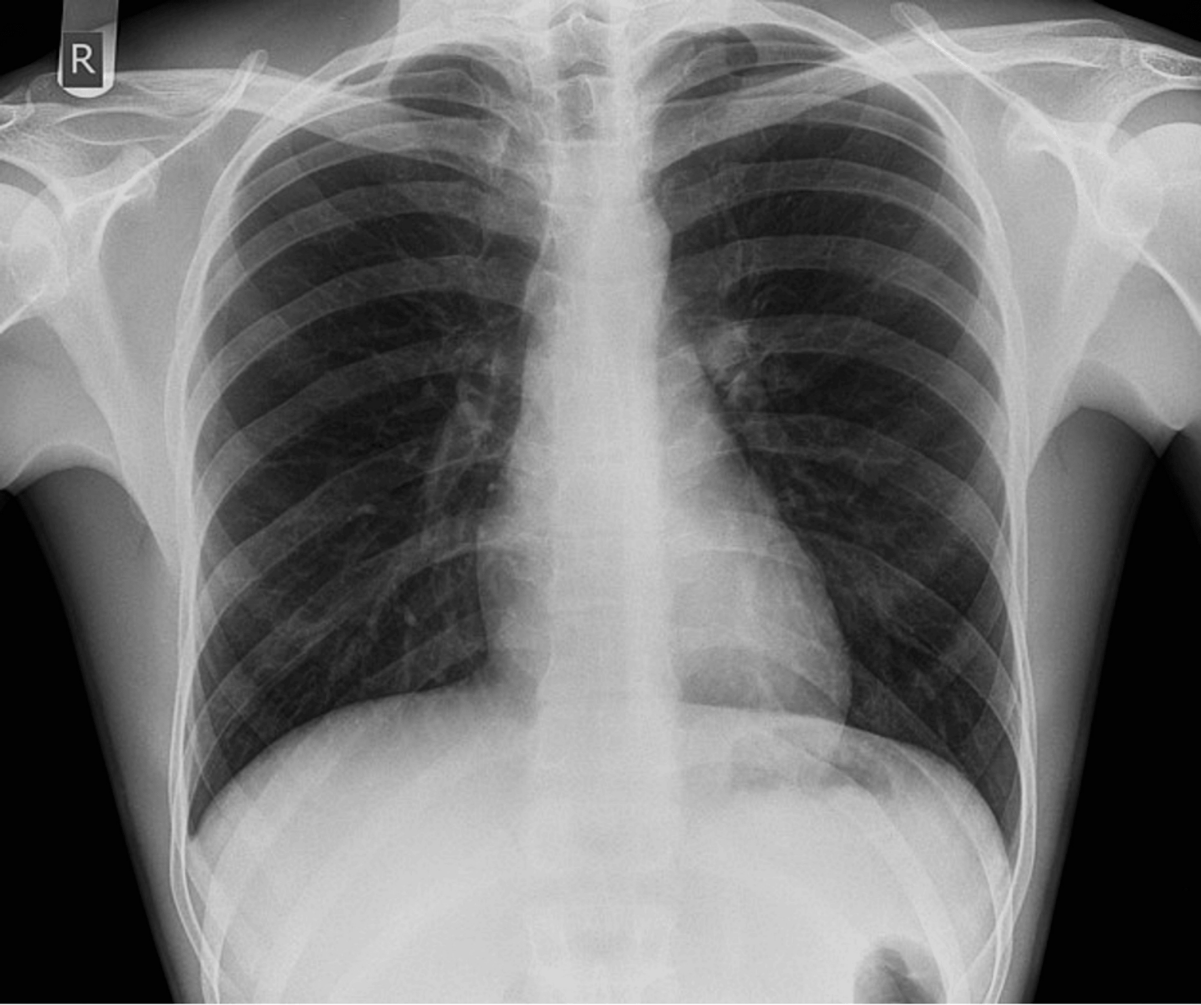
Normal Chest Radiograph at Presentation Patient’s chest X-ray demonstrating no evidence of cardiomegaly, pleural effusions, or pulmonary congestion.
